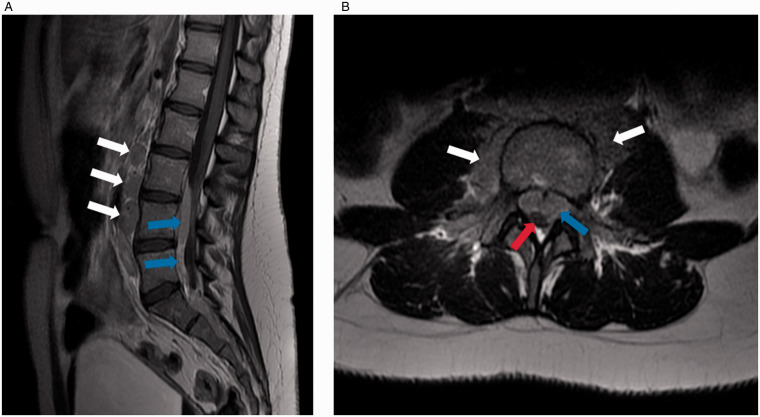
Representative MRI imaging of the epidural mass causing spinal cord compression. A, Sagittal T1 weighted contrast imaging demonstrating the anterior epidural enhancing mass in the lumbar canal (blue arrows) with enlarged paraaortic lymph nodes (white arrows). B, Axial T2 weighted imaging demonstrating enlarged paraaortic lymph nodes and abnormal paraspinal soft tissue (white arrows) and the anterior epidural mass (blue arrow). The thecal sac (red arrow) is displaced posteriorly and compressed.
